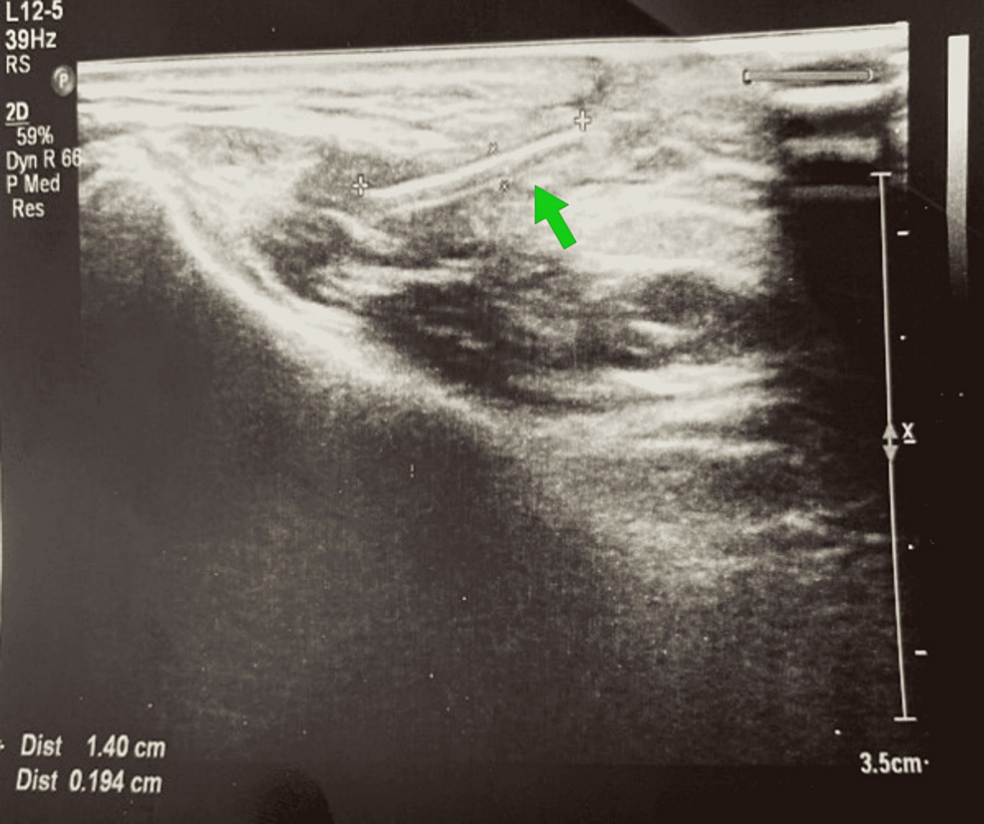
Ultrasonography image of foreign body Subcutaneous, associated with the parotid gland, approximately 14x2 mm in size, linear-shaped, hyperechoic image.
